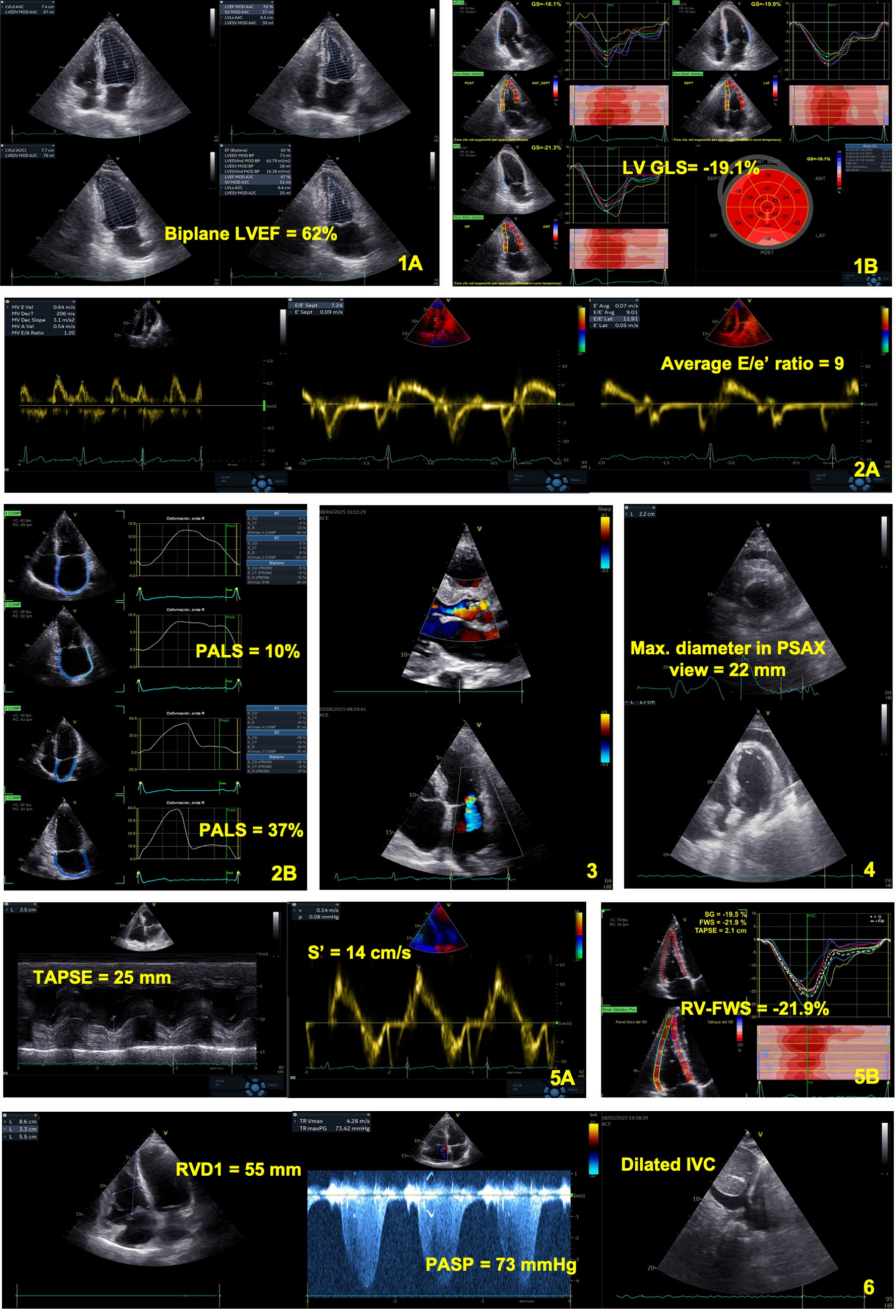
Key echocardiographic acquisitions during oncology patient’s evaluation. 1 Left ventricular systolic functionassessment by traditional Simpson biplane method (1A) and speckle tracking-derived global longitudinal strain (1B). 2Left ventricular diastolic function assessment by traditional methods (E/e′ ratio in 2 A) and left atrial strain (2B). 3 Aorticregurgitation (3A) and mitral regurgitation (3B) in patients undergone chest radiotherapy. 4 Right ventricular systolicfunction assessment by traditional methods (TAPSE and S′ wave velocity in 4 A) and speckle tracking echocardiography(4B). 5 Echocardiographic signs of pulmonary embolism in a cancer patient. 6 Pericardial effusion in a cancer patient
